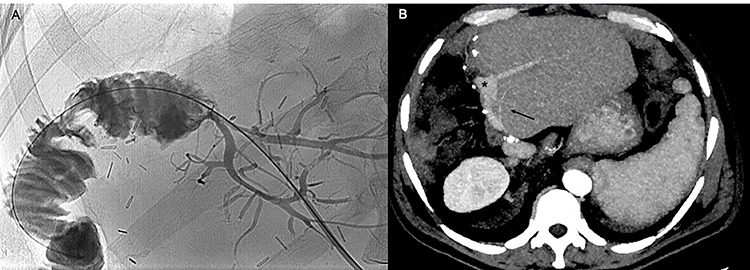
The cholangiogram performed 1 month after the hepatic resection (A) and the CT scan performed 5 months later (B) show regular patency of the bilio-enteric anastomosis, absence of biliary leaks and patency of the portal graft, the left portal branches (black asterisk) and left hepatic artery (black arrow), without signs of local recurrence.
